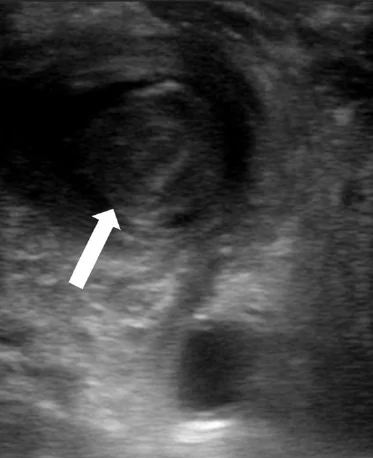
Close-up ultrasound image highlighting the internal jugular vein thrombus (arrow) in a patient diagnosed with Lemierre syndrome.
